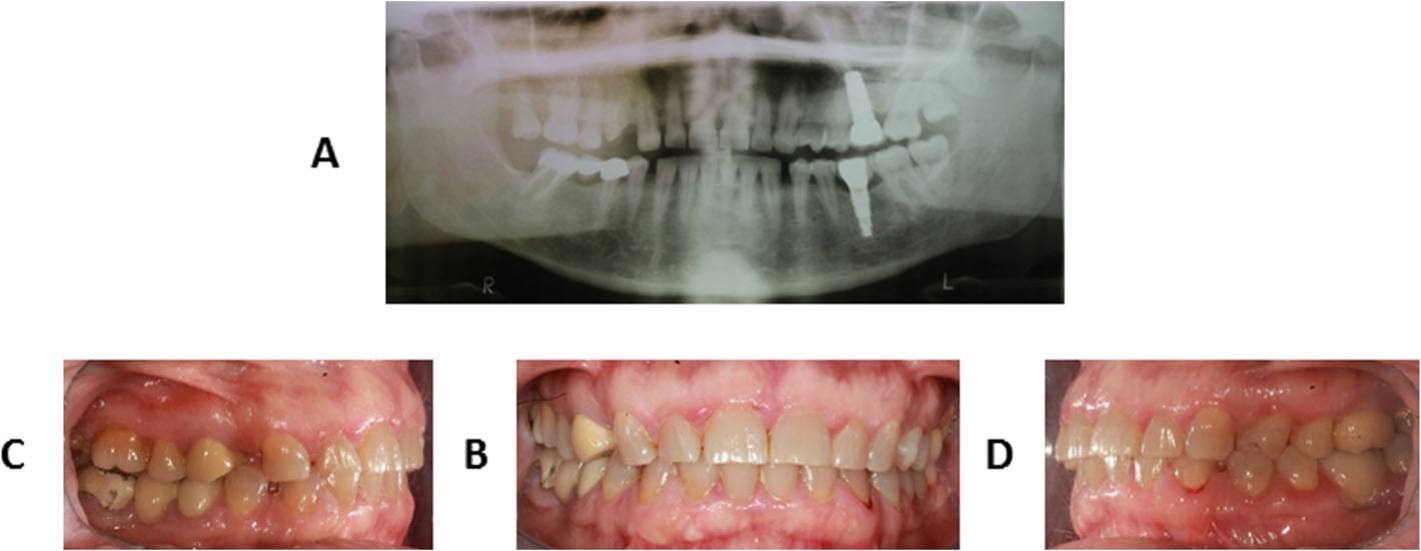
Clinical records of the mother’s proband: panoramic radiographic analysis (a), intraoral frontal view (b), intraoral right lateral view (c), intraoral left lateral view (d)
